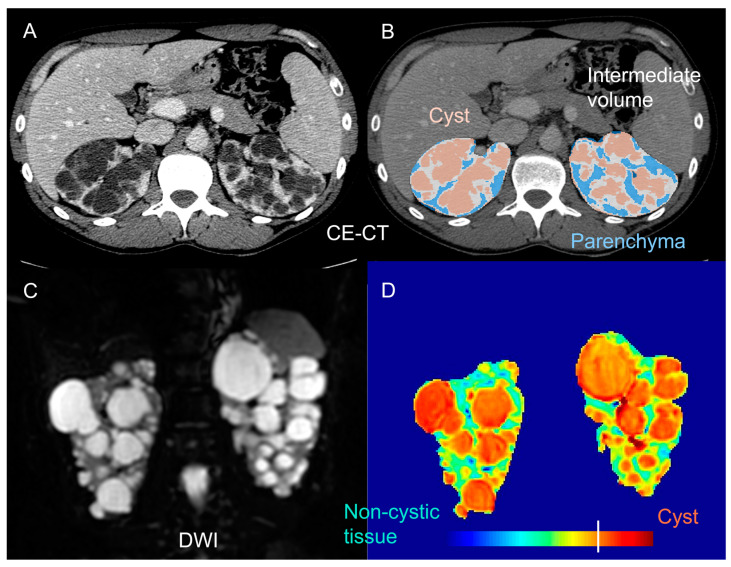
Examples of imaging techniques allowing the quantification and characterization of non-cystic kidney tissue in ADPKD. Top row: the automatic segmentation of cysts, intermediate volume (non-cystic fibrotic component), and residual parenchyma (B) on a contrast-enhanced (CE) CT scan (A), as described in [71]. Bottom row: DWI b0 scan (C) and pertinent DWI-based diffusivity (D) map (D), clearly distinguishing cysts and non-cystic tissue, and allowing the characterization of the non-cystic component, as described in [75].
